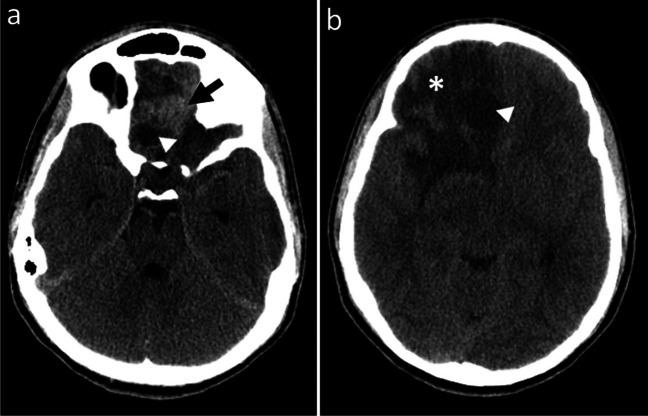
Axial non-contrast computed tomography images of the brain in a 16-year-old boy show a right frontobasal lesion. a Slice at the frontobasal level shows a solid hyperdense portion (*arrow*) and cystic component (arrowhead). b Slice at a higher level reveals the cystic component (arrowhead), accompanied by extensive vasogenic edema (asterisk) in the right frontal region
